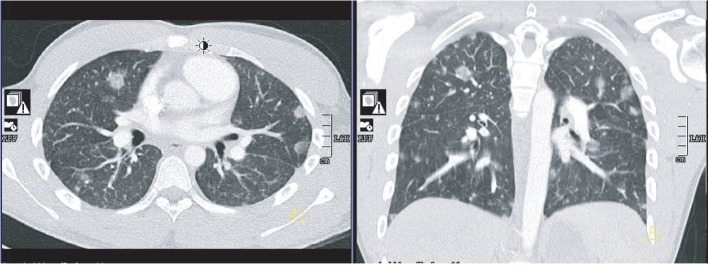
Chest computed tomography (CT) on admission showing bilateral pulmonary nodules consistent with septic pulmonary embolization (SPE) in the setting of Methicillin-Resistant Staphylococcus aureus (MRSA) bacteremia.
